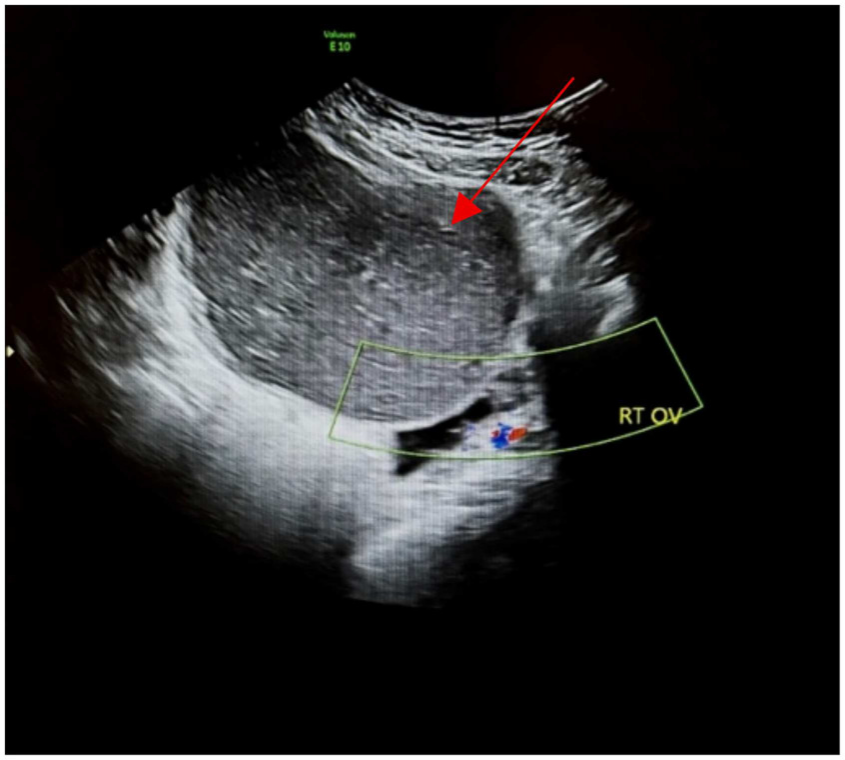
Transabdominal ultrasound of the right ovary (RT OV) demonstrates homogeneous hypoechoic cystic adnexal mass without internal increased vascularity (red arrow).
